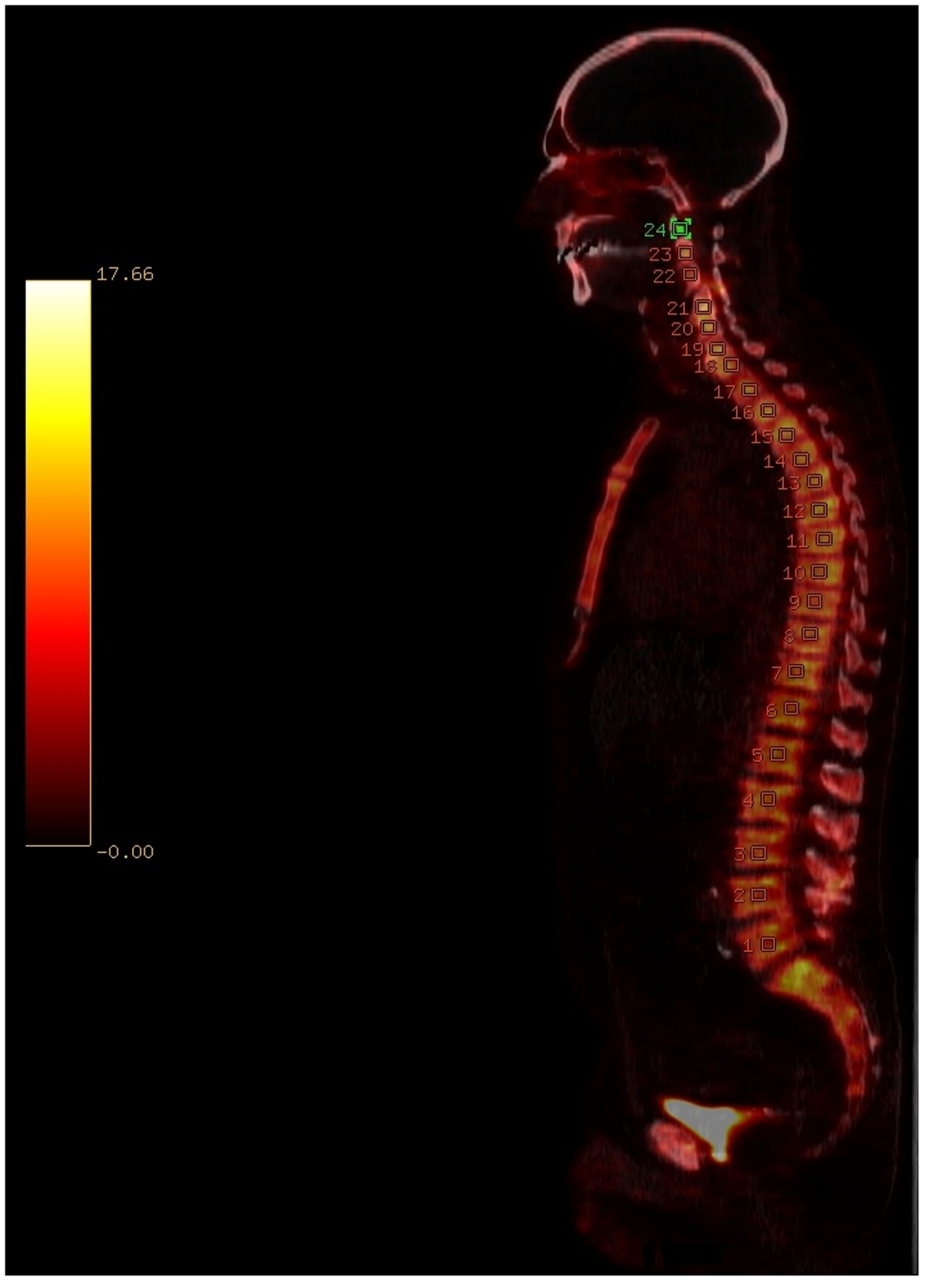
A fixed-size ROI (826 mm^3^) was placed on the vertebral bodies (C1-L5) to measure the SUV_max_ values of the spine.
